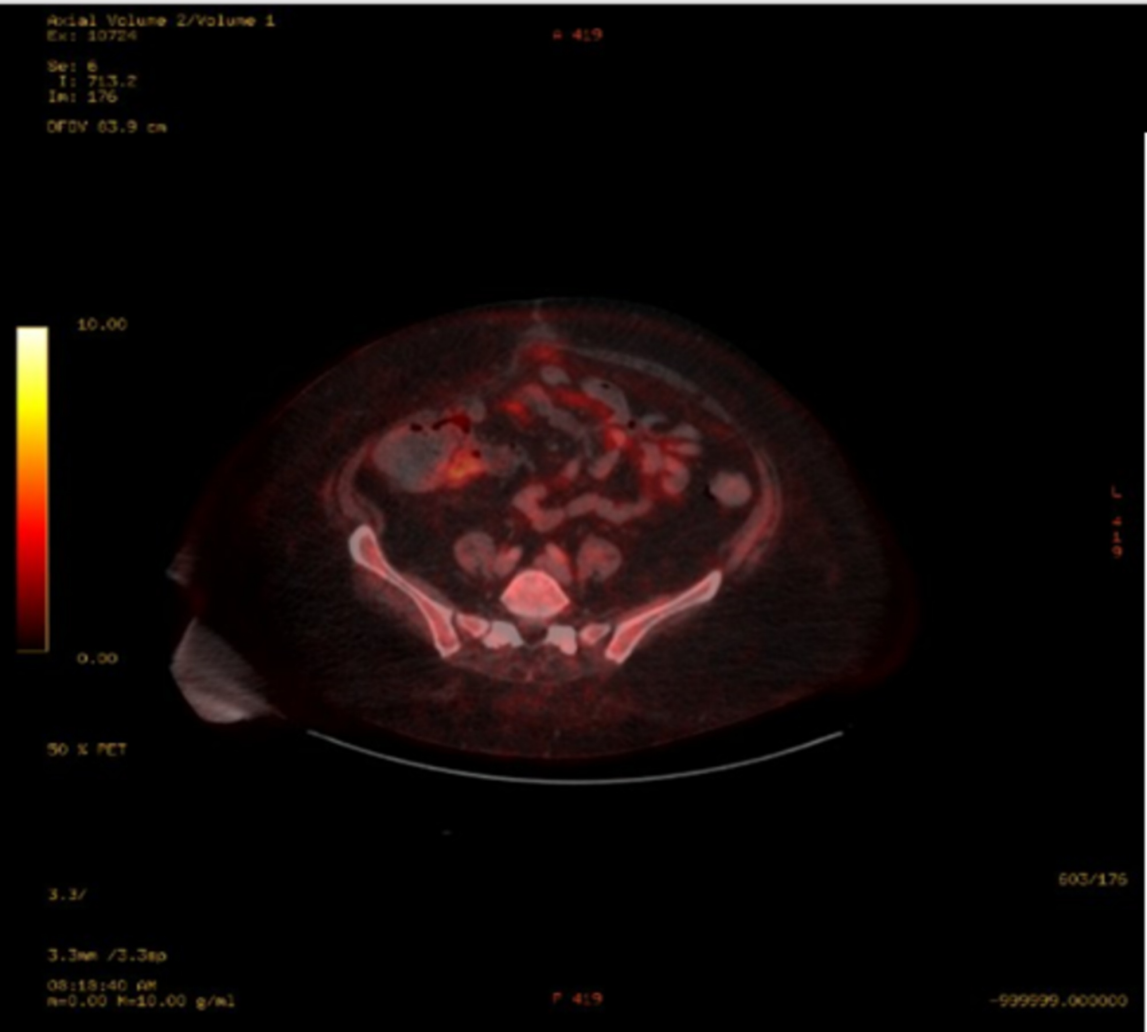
Repeat PET-CT - No evidence of recurrent malignancy or metastatic disease. PET-CT: positron emission tomography-computed tomography
